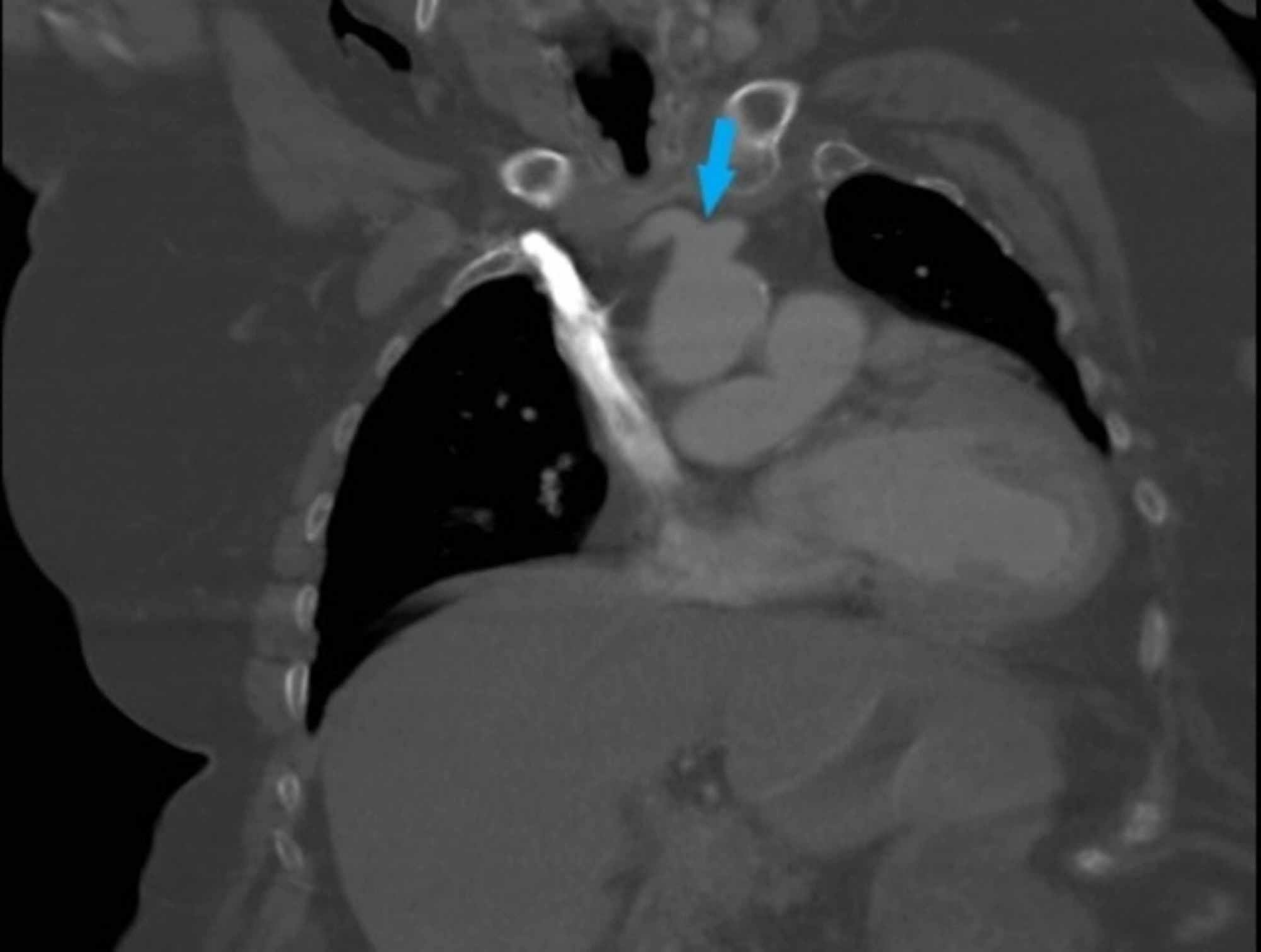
Chest computed tomography (CT), coronal view, showing a bicarotid trunk (arrow).
